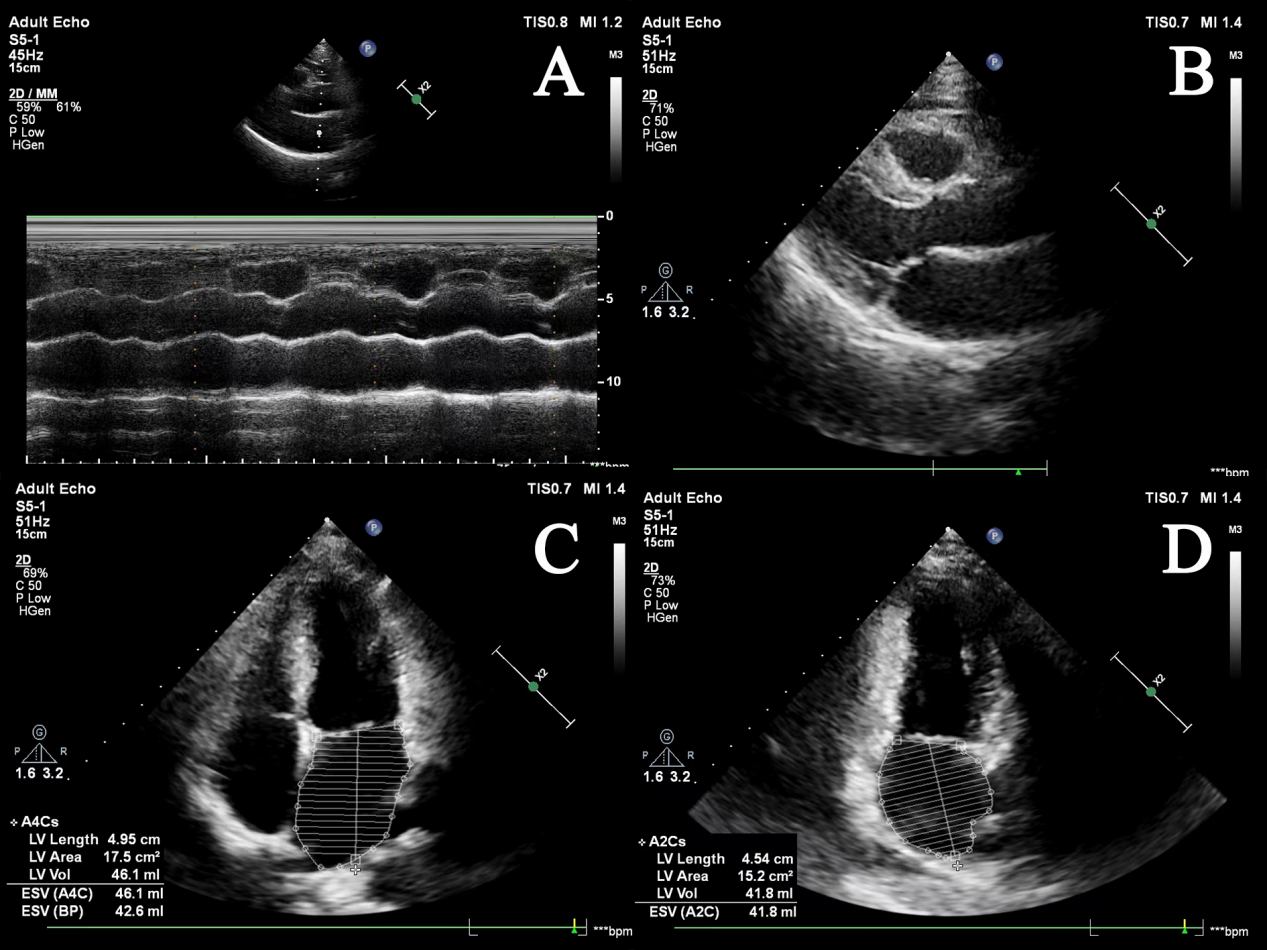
Cardiac echocardiography images of the left atrium. (A) M-mode
